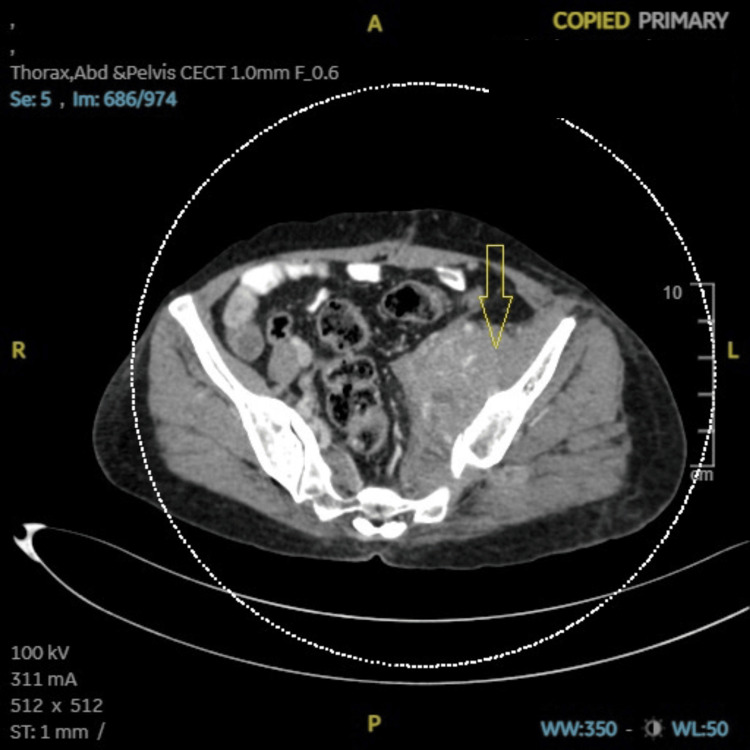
Case 1: CECT (abdomen and pelvis) showing left pelvic nodal mass (9 × 10 × 6.5 cm) along iliac vessels encasing left external iliac artery, infiltrating left iliopsoas, obturator internus muscle (arrow). CECT: contrast-enhanced computed tomography, A: anterior, R: right, L: left, P: posterior, ST: soft tissue, WW: window width, WL: window level.
